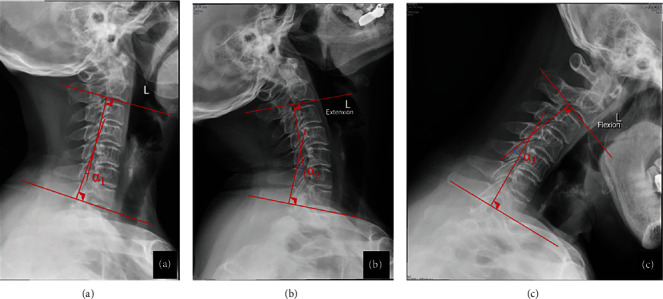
Radiological measurements: (a) the measurement of Cobb angle (*α*_1_) on the neutral position lateral X-ray; (b, c) the measurement of cervical range of motion (ROM) on the extension and flexion lateral X-ray, ROM = *α*_2_+*α*_3_.
